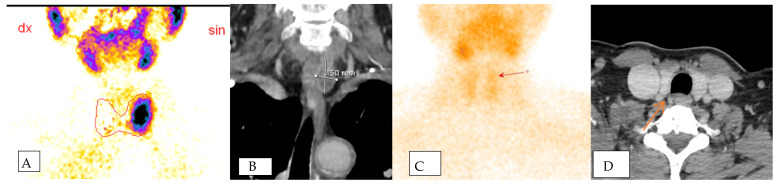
The figure shows positive scintigraphy (A) and CT scan (B) of the left sided parathadenoma in one patient which localization was confirmed using SVS and postoperative material. Figure (C,D) shows mismatch between two radiological non-invasive methods. Scintigraphy (C) shows suspected left-sided parathadenoma, while CT scan (D) shows right-sided parathadenoma. SVS and operative material confirmed left-sided parathadenoma in this patient.
